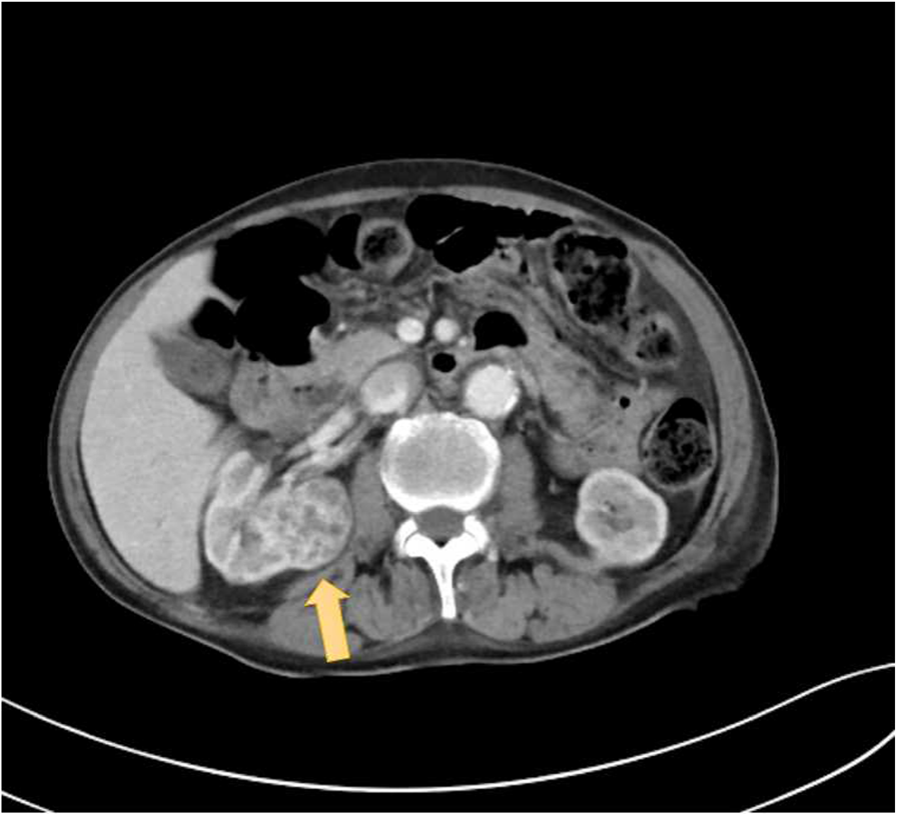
Axial view of contrasted computed tomography scan of the abdomen shows a heterogeneously enhancing right renal mass (*orange arrow*)
